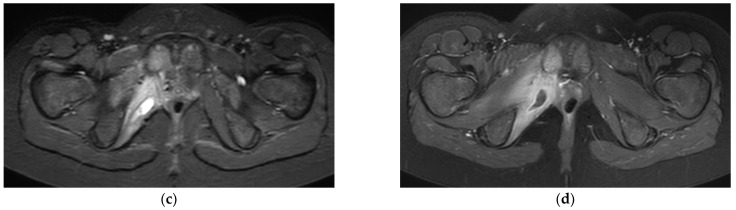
A 5-year-old boy with pyomyositis of the right obturator muscles and osteomyelitis of the right hip bones. (a) Soft tissue ultrasound and (b) hip X-ray are negative. (c) Axial fat suppressed T2-weighted magnetic resonance (MR) image shows muscle edema and an abscess in the obturator internus muscle. On (d) fat suppressed axial T1-weighted post-contrast image the abscess shows hypointense fluid collection with rim enhancement. MR images show hyperintensity of the right pubic bone (osteomyelitis).
